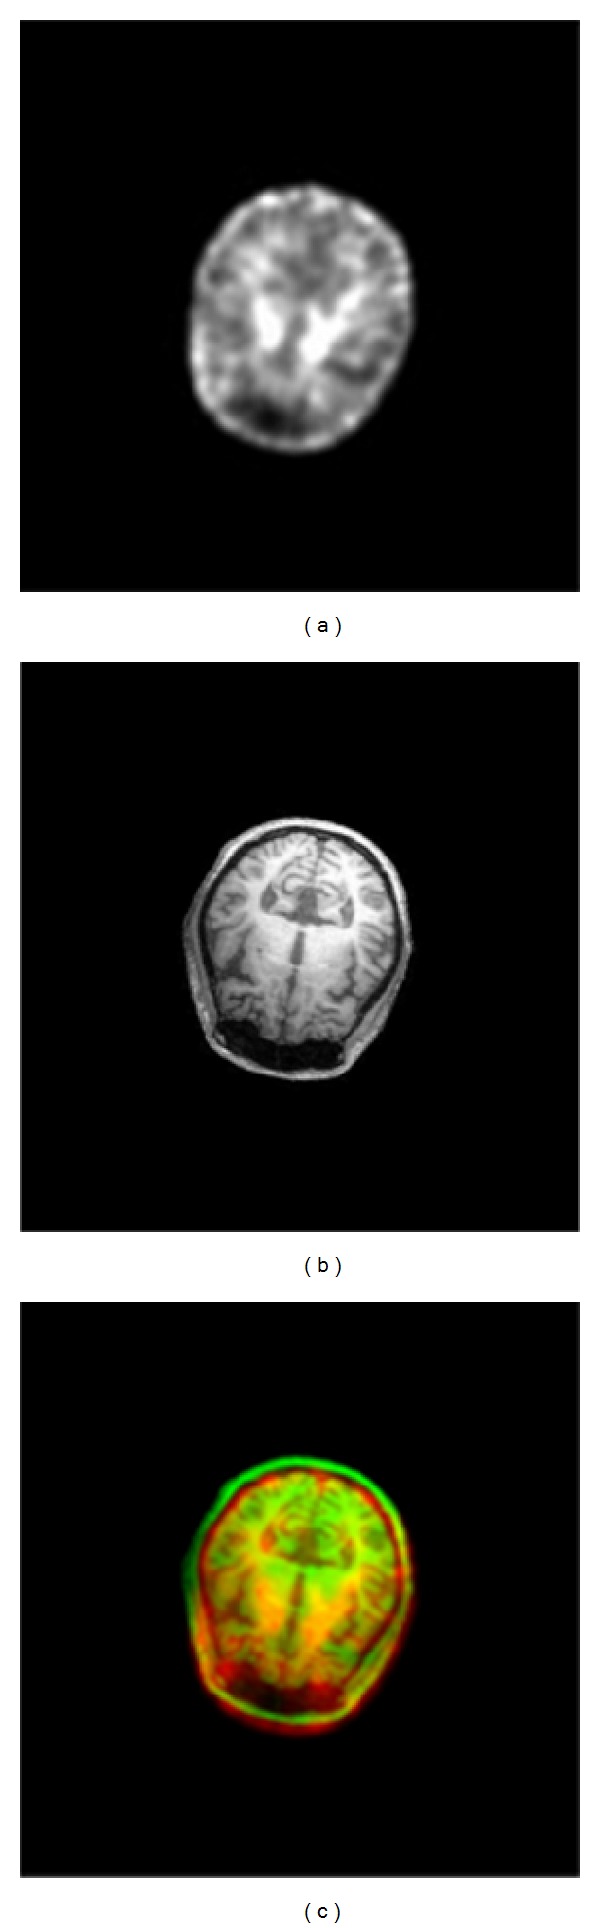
Registration between (a) SPECT and (b) MR to give the final overlaid image in (c).
